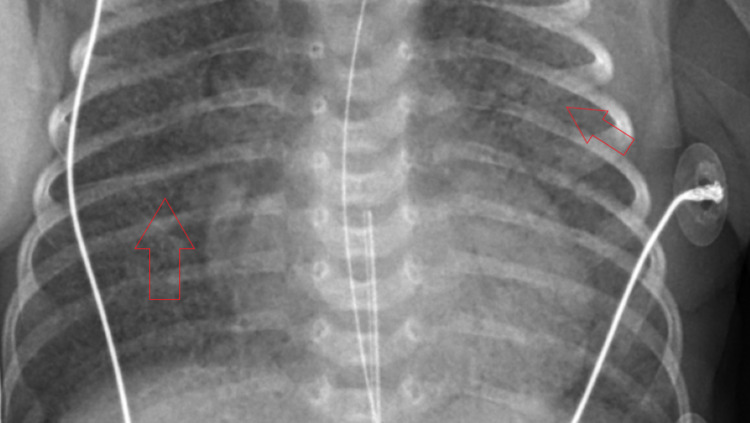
CXR on day 6 of life display granular infiltrates, consistent with hyaline membrane disease (arrows) CXR - chest x-ray
